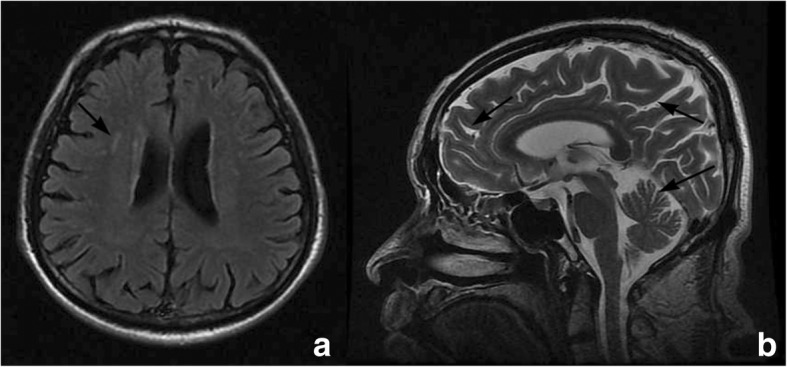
MRI showed a demyelination in right corona radiata (arrow in 2a), with mild cortical and cerebellar atrophy (arrows in 2b)
